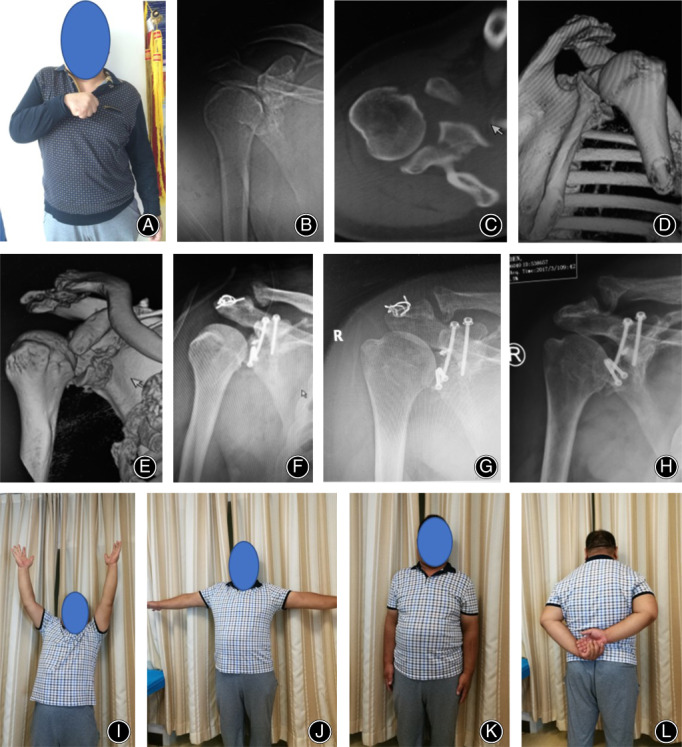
A 49 years old man with glenoid and acromion fractures result to right chronic anterior shoulder subluxation more than 60 days (No.13 patient). (A): This male patient complained severe pain and limited activity. (B): Preoperative anteroposterior radiograph showing a chronic anterior shoulder subluxation. (C‐E): Preoperative CT scans showing chronic anterior subluxation with glenoid and acromion fractures. (F): Anteroposterior radiograph postoperatively showing right shoulder reduction with ORIF of glenoid and acromion factures, coracoid graft was fixed back to scapula with two screws. (G): Anteroposterior radiograph showing right shoulder reduction 1 year postoperatively before the wire tension bend in acromion removing. (H): Anteroposterior radiograph showing right shoulder after the wire tension bend removing. (I‐L): The right shoulder function of this patient basically returned to normal 1 years after surgery.
